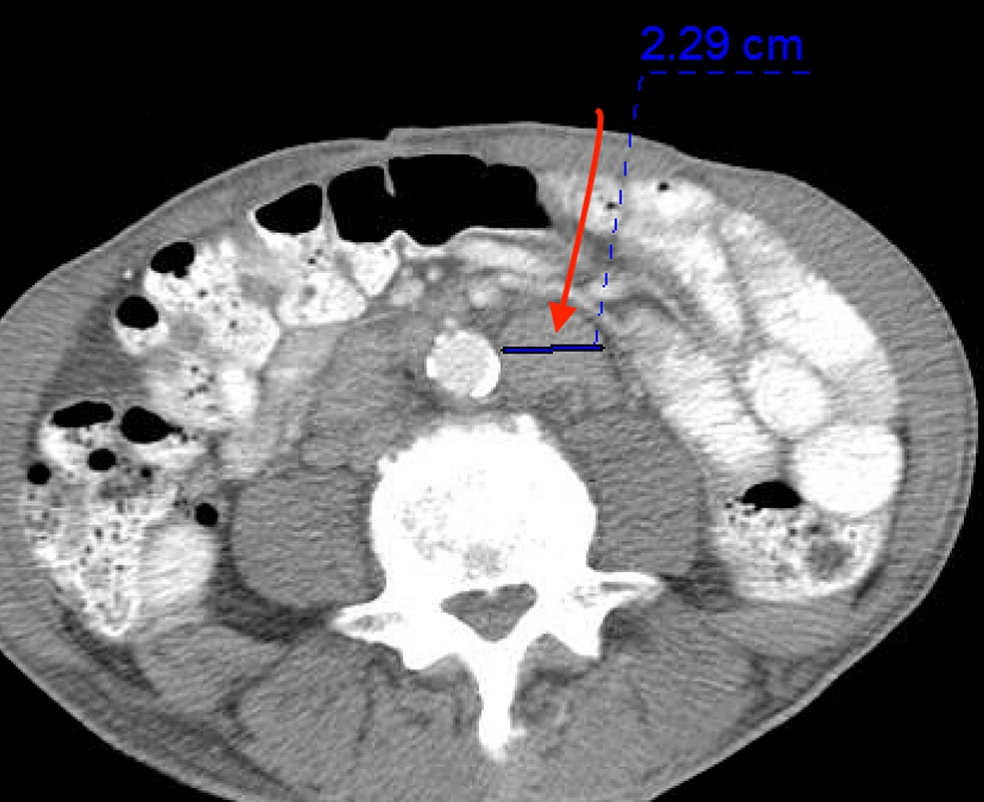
The arrow shows an enlarged lymph node on CT scan of the abdomen.
